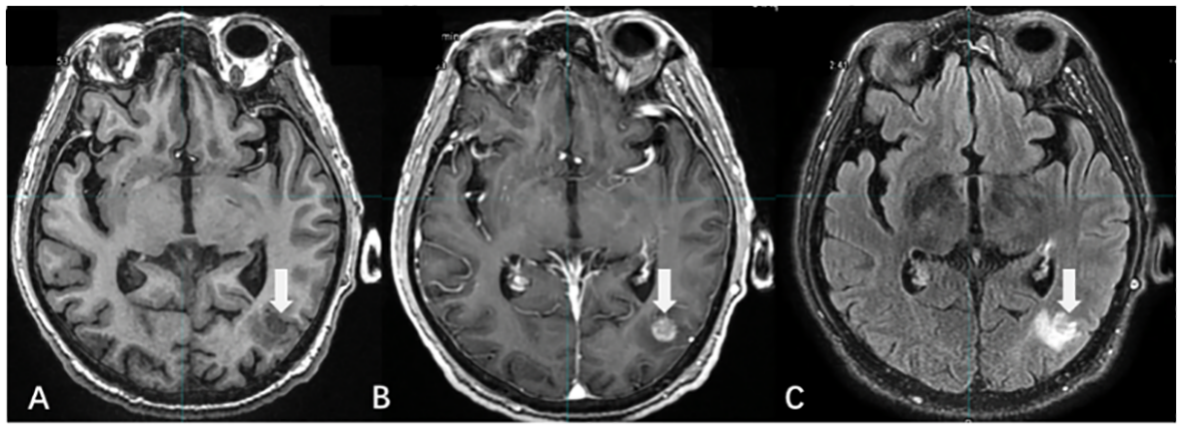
The display differences of BMs among T1WI, T1WI+C, and T2/FLAIR. [(A) T1WI without contrast; (B) CE-T1WI; (C) CE-T2/FLAIR].
